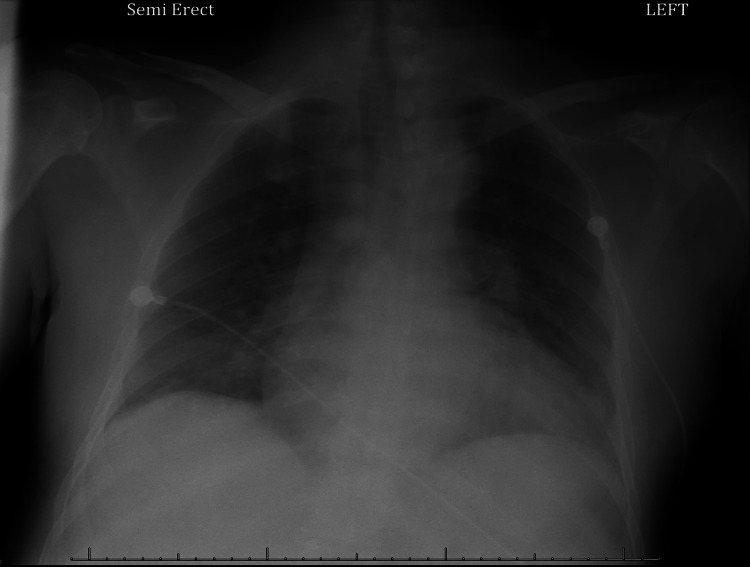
Chest X-ray showing cardiomegaly with mild interstitial pulmonary edema.
